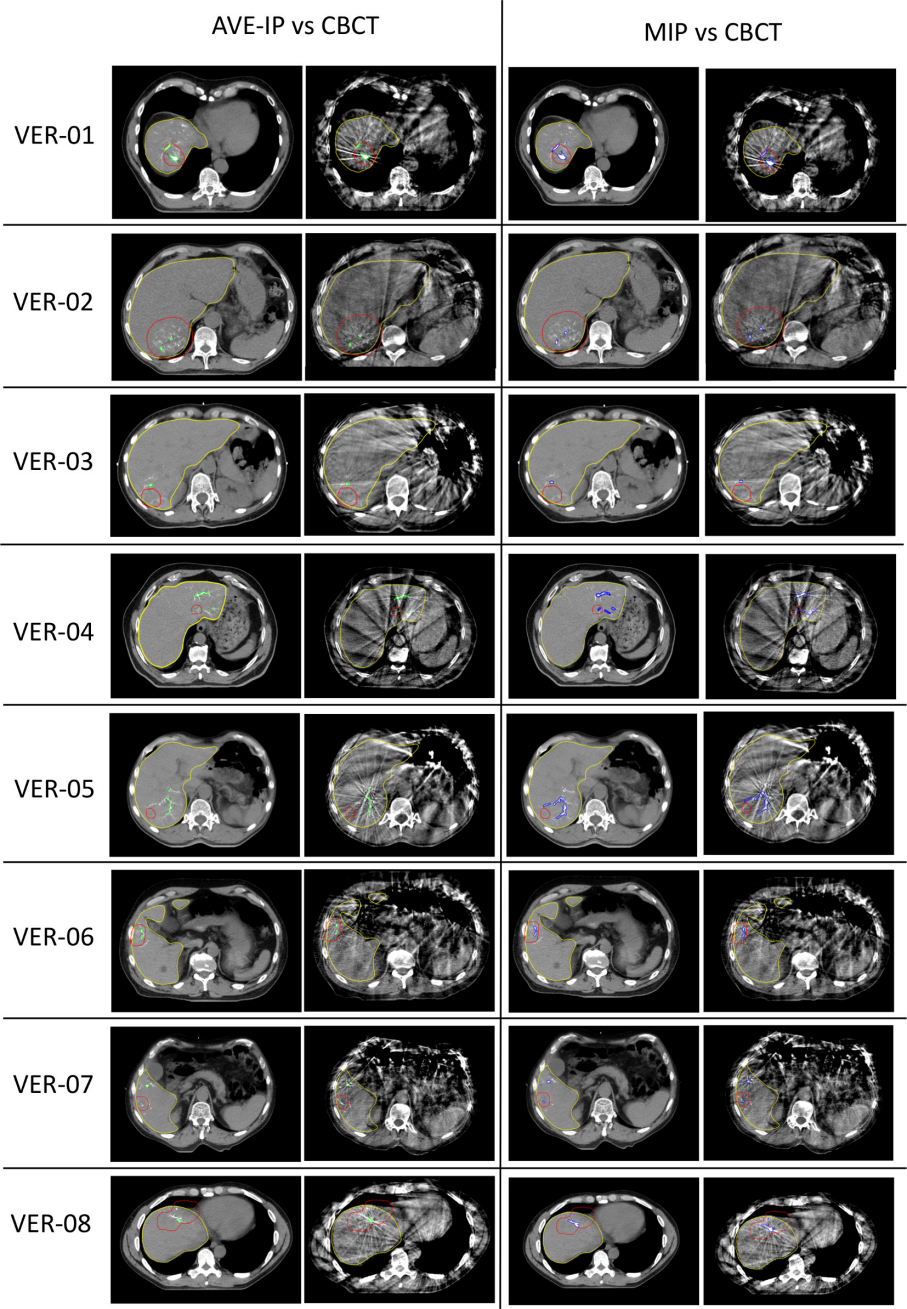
Matching of RO beads on AVE-IP, MIP and CBCT. Code: Red, planning target volume; Yellow, liver contour; Green, RO beads contoured on AVE-IP at 150 HU and matched to CBCT; Blue, RO beads contoured on MIP at 250 HU and matched to CBCT
